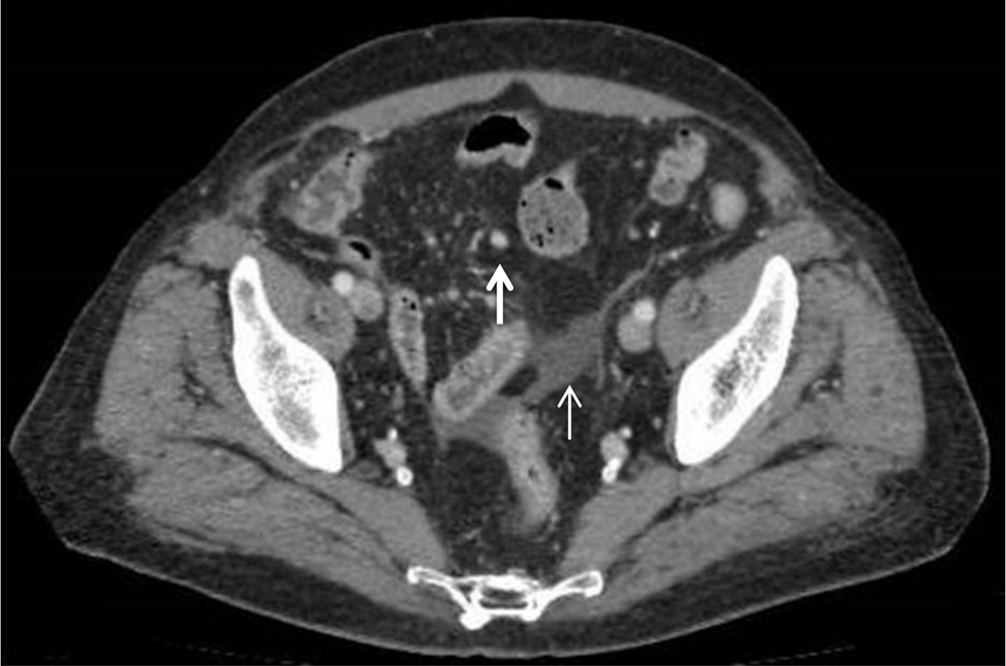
Abdominal CECT. Mesenteric lymph nodes (arrow) and pelvis ascites (thin arrow). CECT, contrast enhanced CT.
